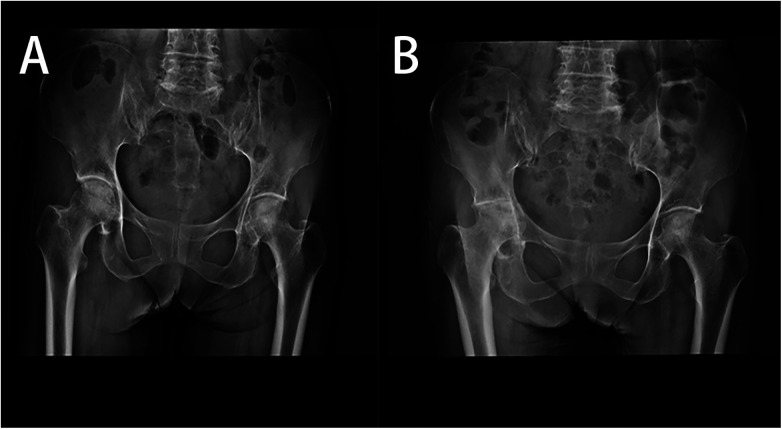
(A) 2023-07-14 Bilateral hip anteroposterior x-ray; (B) 2023-08-24 bilateral hip anteroposterior x-ray.
